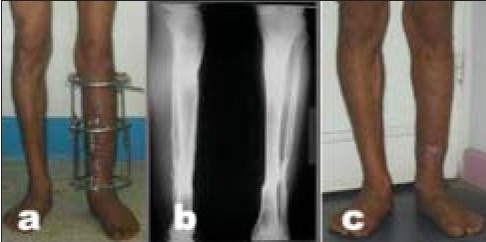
(a) Clinical photograph during treatment, the patient was allowed weight-bearing. (b) Follow-up radiographs (lateral and anteroposterior) showing consolidation of the nonunion and limb lengthening of 7 cm. (c) Clinical photograph showing equal limb length.
